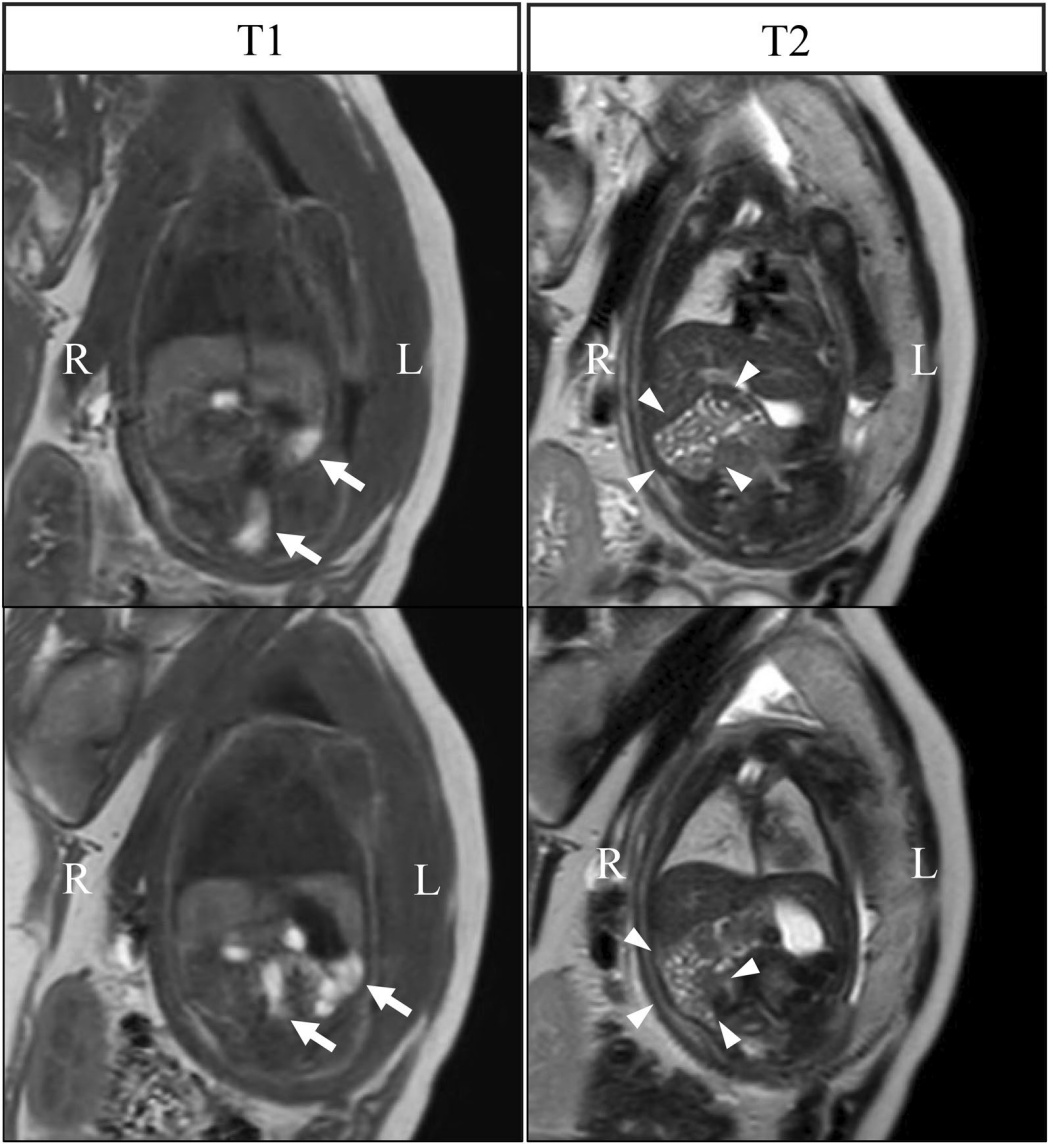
Fetal magnetic resonance imaging. The colon, visualized as hyperintense in the T1-weighted image, was distributed in the left hemi-abdomen (arrow). The small intestine, visualized as hyperintense in the T2-weighted image, was distributed in the right hemi-abdomen (arrowhead)
